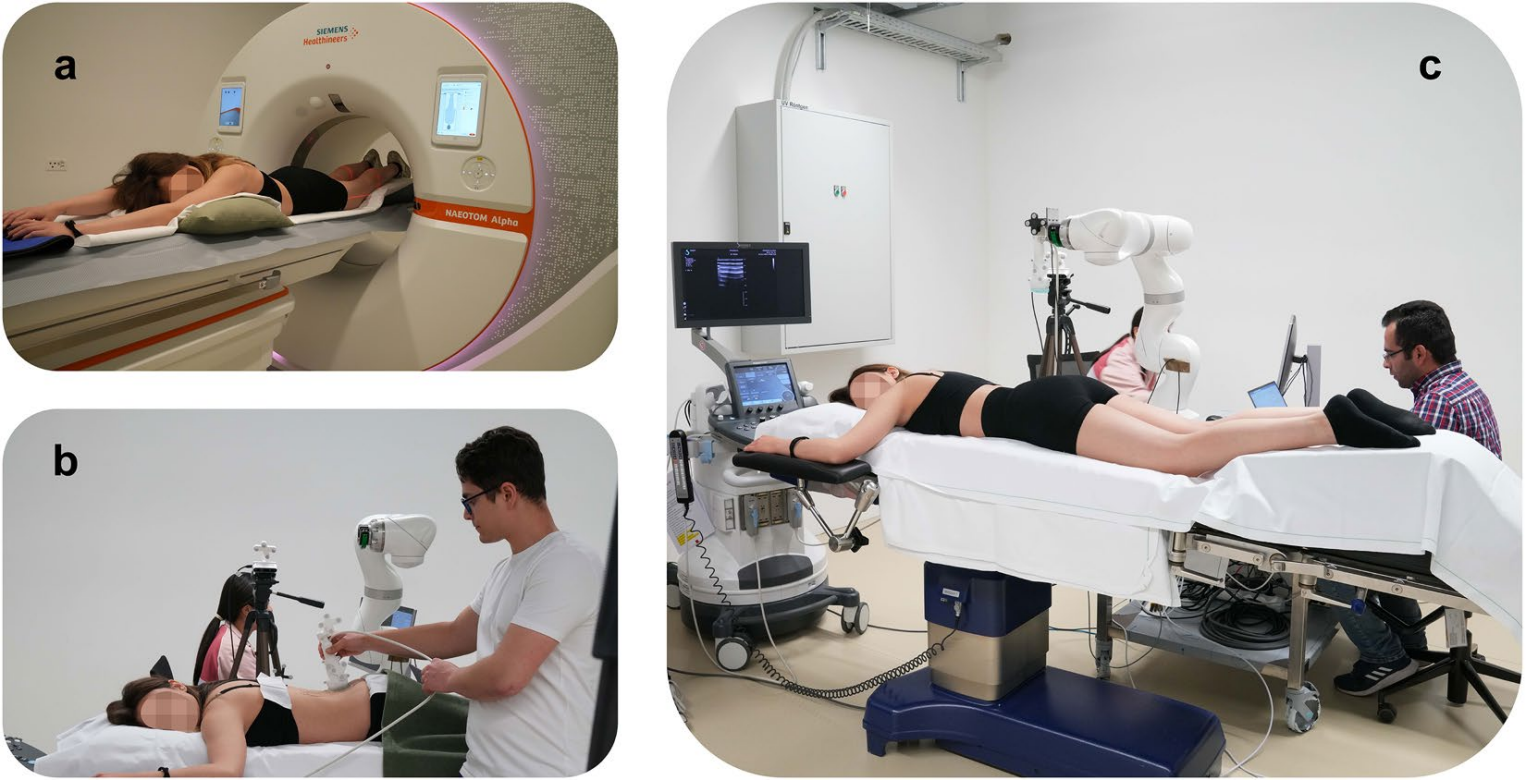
Experimental CT, HUS, and RUS scan setup. The volunteers were positioned prone on the table during (a) CT Scan, (b) HUS, and (c) RUS. In all three imaging examinations, the arms were rested above the head, and a positioning cushion supported the feet and lower legs for comfort. The US device was positioned at the head of the volunteer. The HUS and RUS scans were conducted in the same tracking space, with the camera placed approximately two meters away on the left side of the volunteer.
